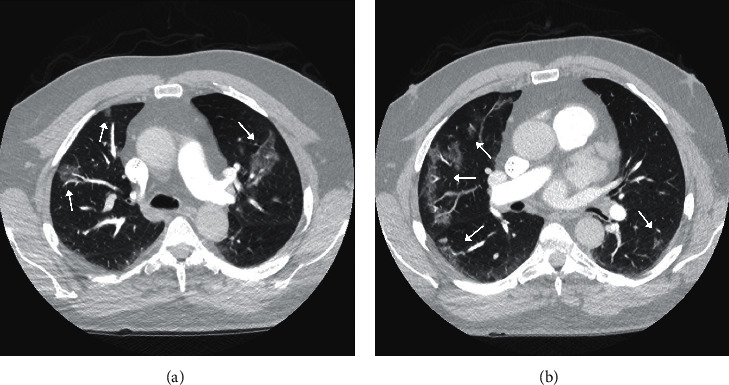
A 67-year-old male with a past medical history of sudden cardiac arrest with the placement of a defibrillator and a recent travel history to Europe presented with nonproductive cough, myalgia, and fever. (a, b) The CT pulmonary arteriogram was performed to rule out pulmonary embolism and demonstrated multiple patchy, peripheral, more organized ground-glass opacities (arrows) compared to the patient in Figure 1. The patient was tested positive for COVID-19.
